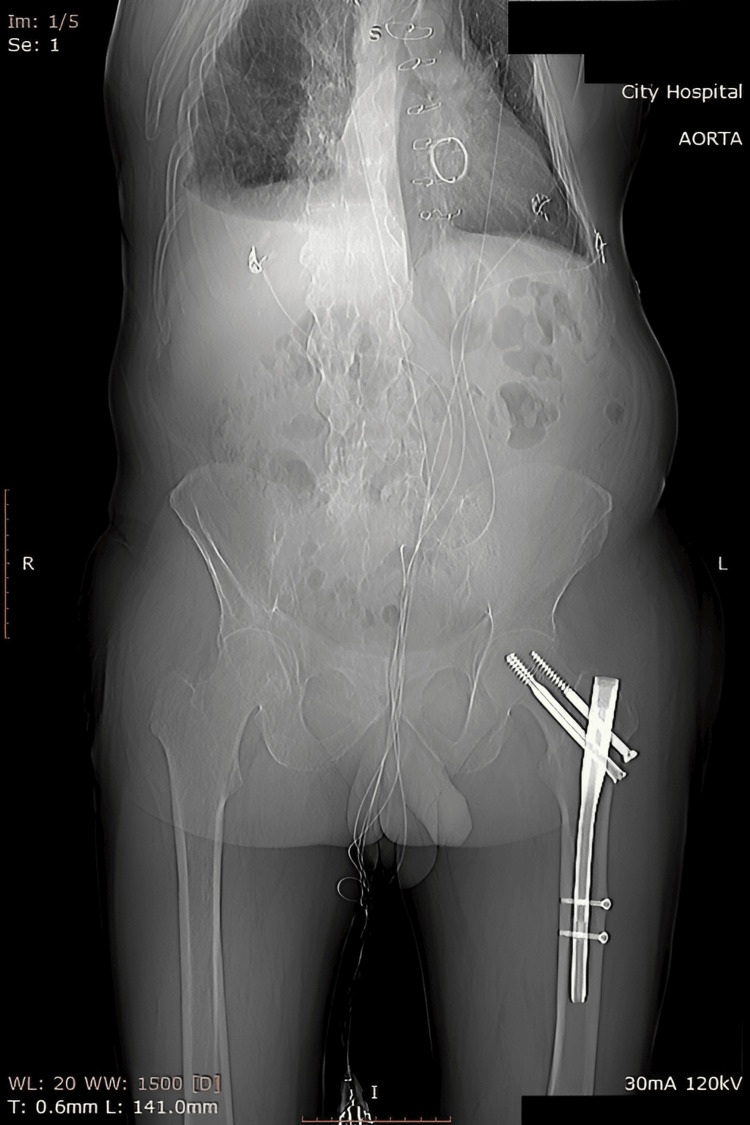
Postoperative X-ray of the patient.
